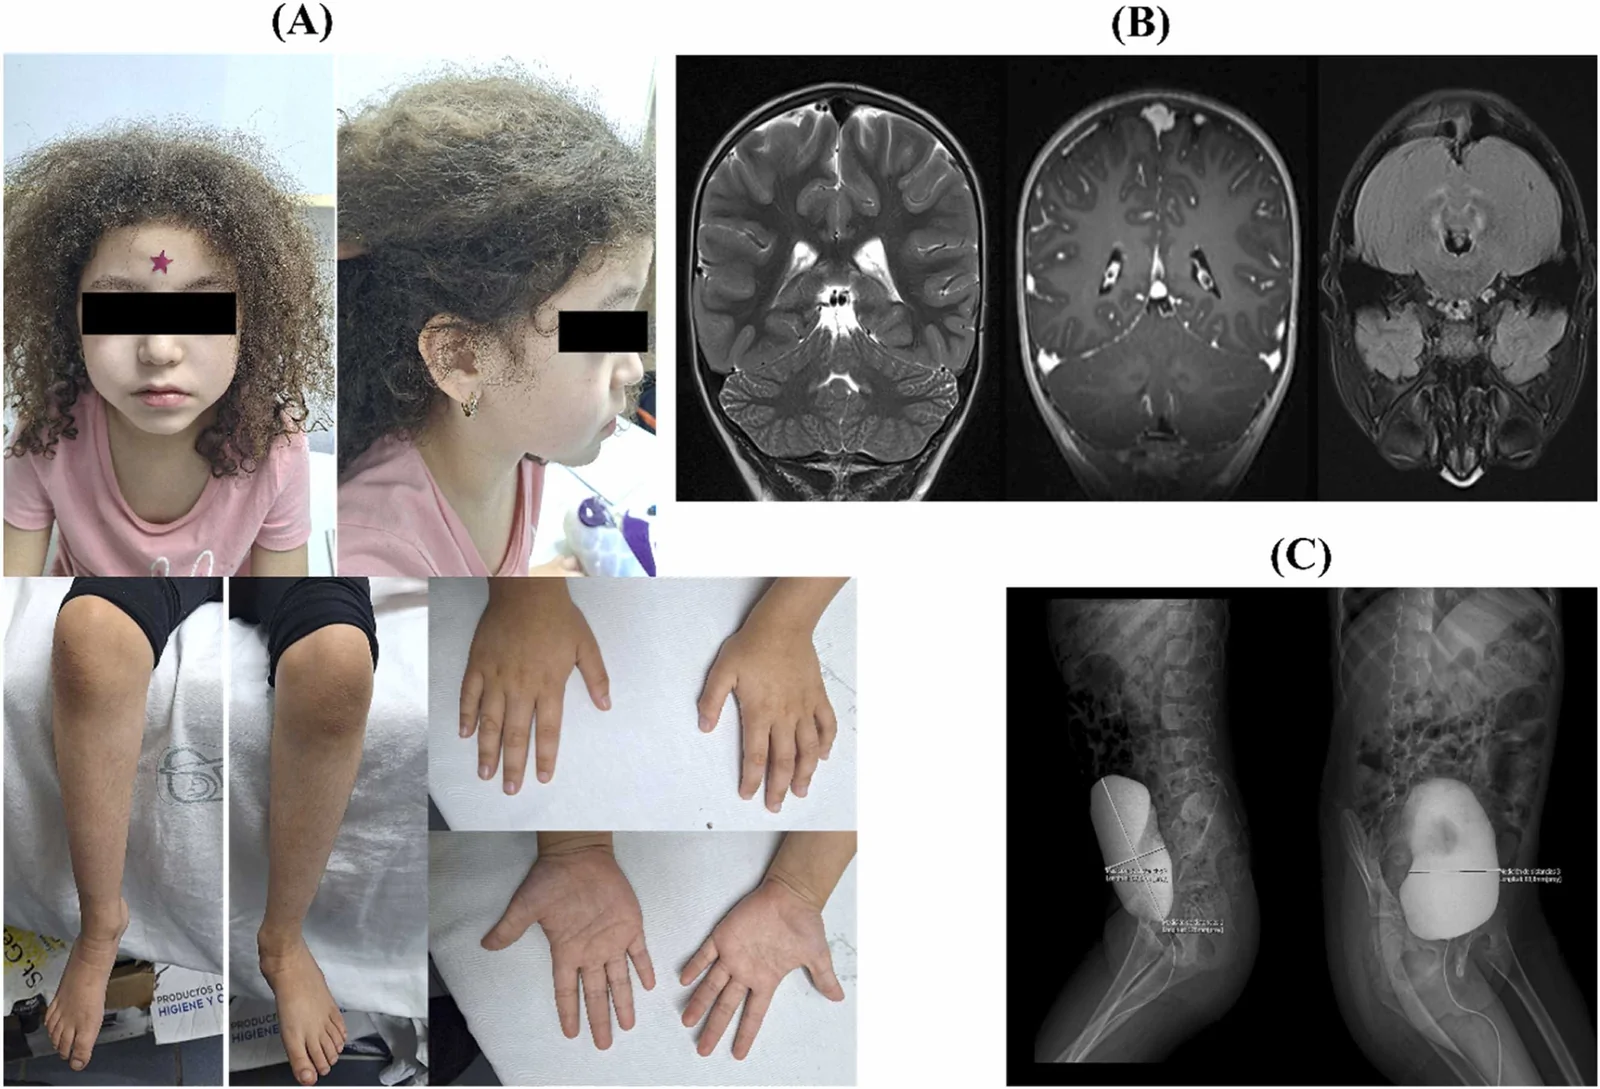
Clinical and radiologic findings. (A) Clinical examination shows dysmorphic facial features, kinky hair, bilateral blepharoptosis, small thenar eminence, and reduced distal limb muscle bulk. (B) Brain MRI – coronal (top) and axial (bottom) views – demonstrating hyperintense signal in the cerebellar white matter surrounding the dentate nuclei, compatible with gliosis or hypomyelination. (C) Contrast cystogram – lateral (left) and anteroposterior (right) projections –showing an enlarged-capacity bladder with extrinsic compression from rectosigmoid contents.
